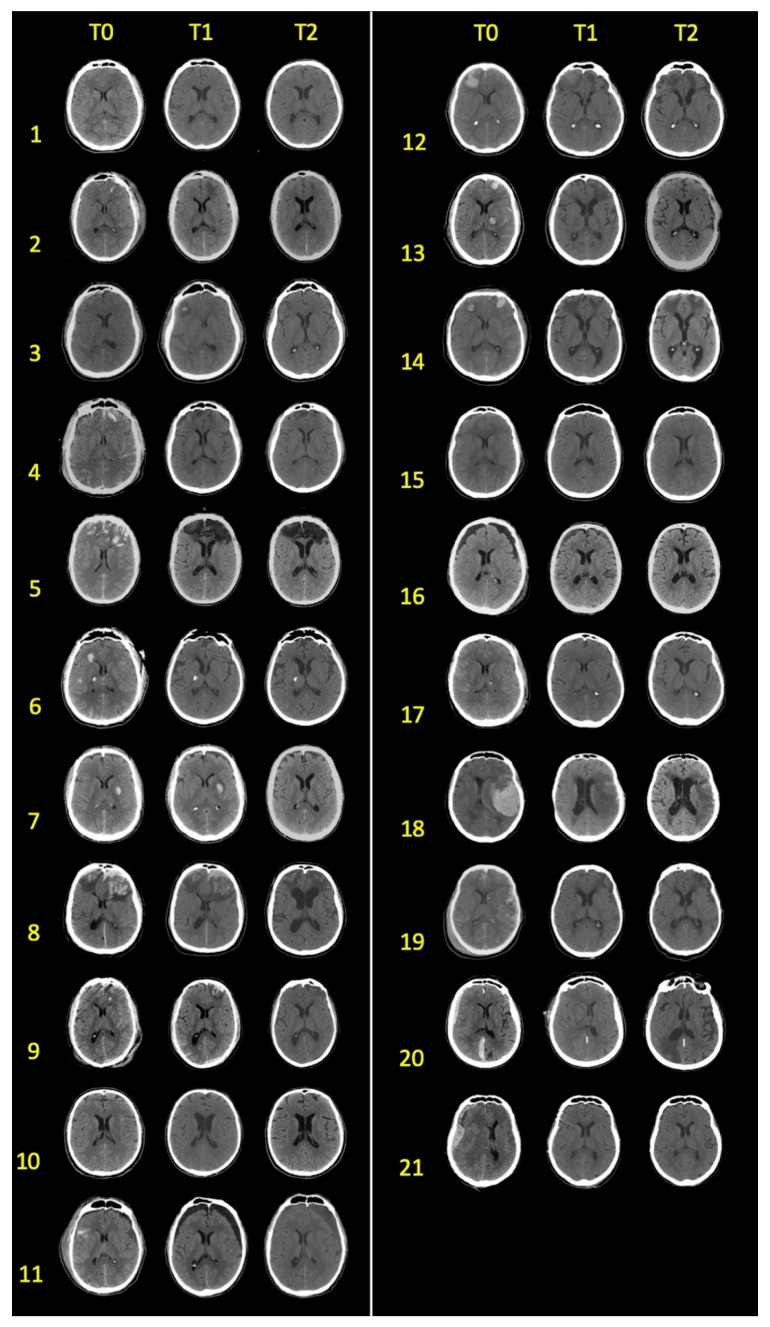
Axial slices of CT scans of each patient collected at 3 (T0), 30 (T1) and 90 (T2) days from injury.
